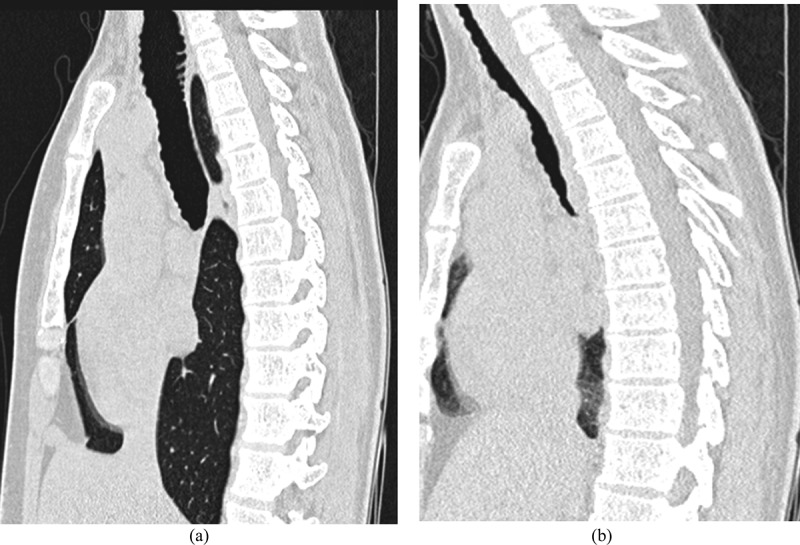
Method 2: paired end-inspiratory (a) and end-expiratory (b) sagittal reconstructed CT images showing maximal collapse of more than 50%.
